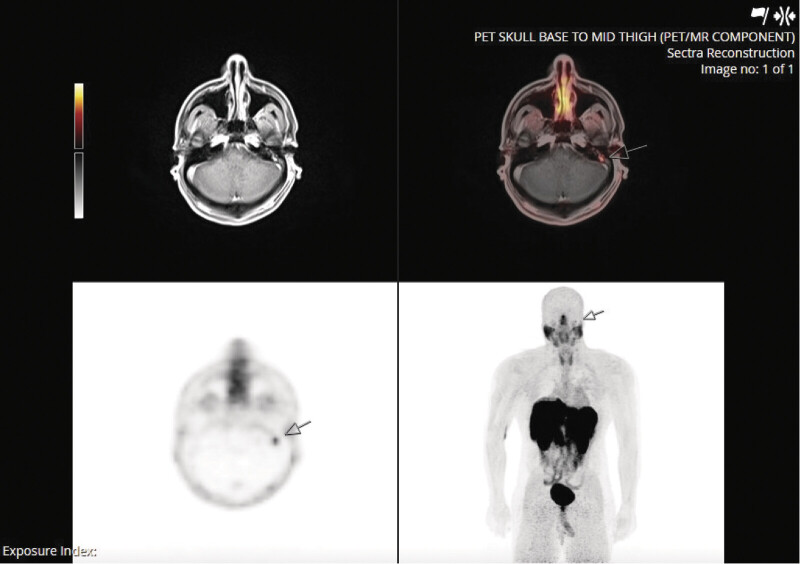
MRI/PET scan. Localized DOTA-TATE avidity (ma SUV 3.5) seen in the left mastoid (arrows) that was suspicious for residual DOTA-TATE avid neoplasm. ma SUV; maximum standardized uptake value.
